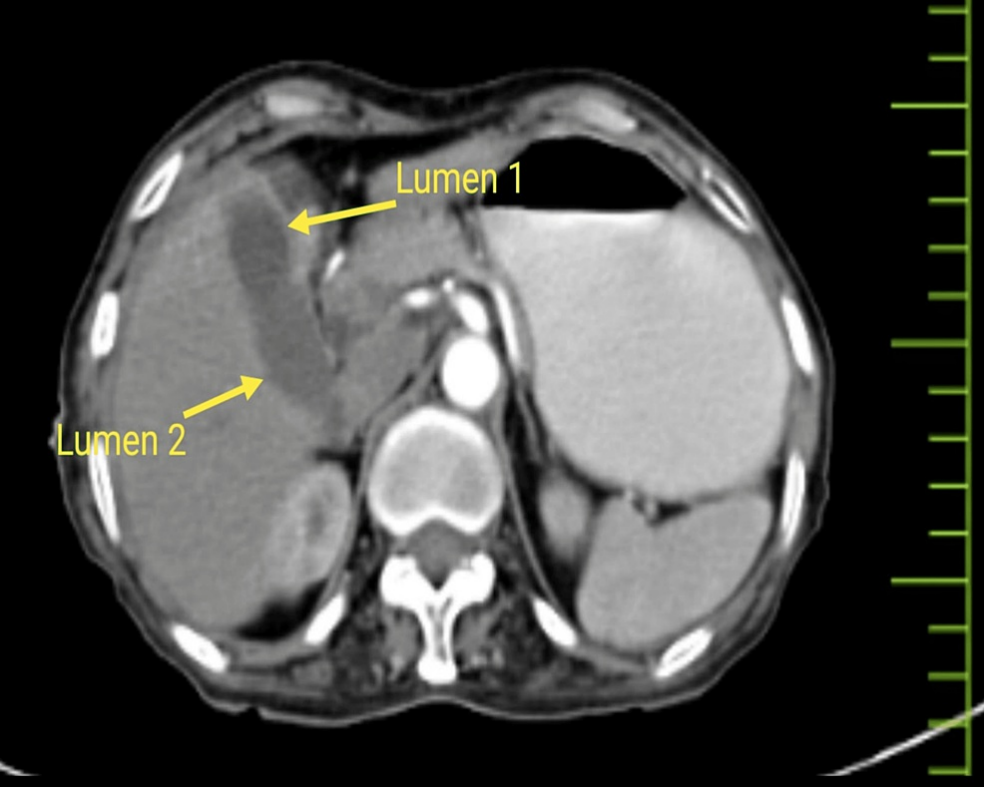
Computed tomography showing abnormally shaped gall bladder
